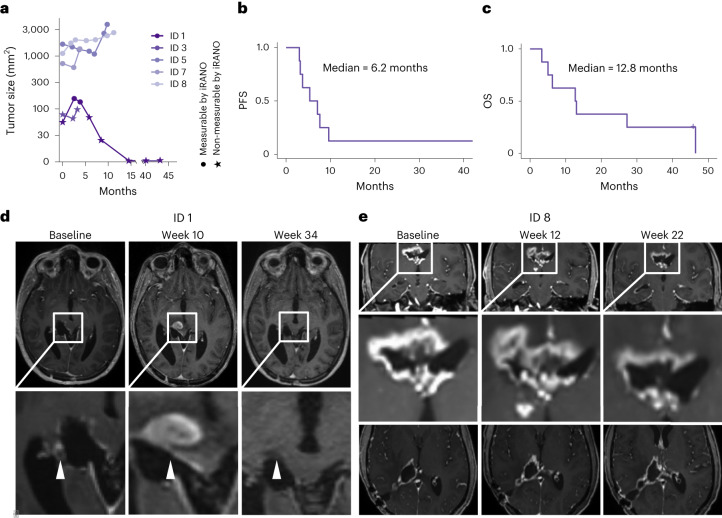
Clinical response to H3K27M-vac. a, Tumor size in mm^2^ as a function of time in months from start of vaccination. Size determined by product of maximal orthogonal diameters on T1-weighted contrast-enhanced MRI imaging. Dots indicate measurements that are considered measurable by iRANO criteria (cerebral lesion with diameter >10 mm). b,c, PFS (b) and OS (c) since the start of vaccination. d, T1-weighted with contrast enhancement (CE) MRI sequences of PsPD of patient ID 1 at baseline, week 10 and week 34. White arrows indicate tumor lesion with PsPD at week 10. e, T1-weighted with CE MRI series of patient ID 8 with early progression between baseline and week 12 followed by disease stabilization concurrent to first detectable H3K27M-specific immune response in peripheral blood in week 18. Source data
